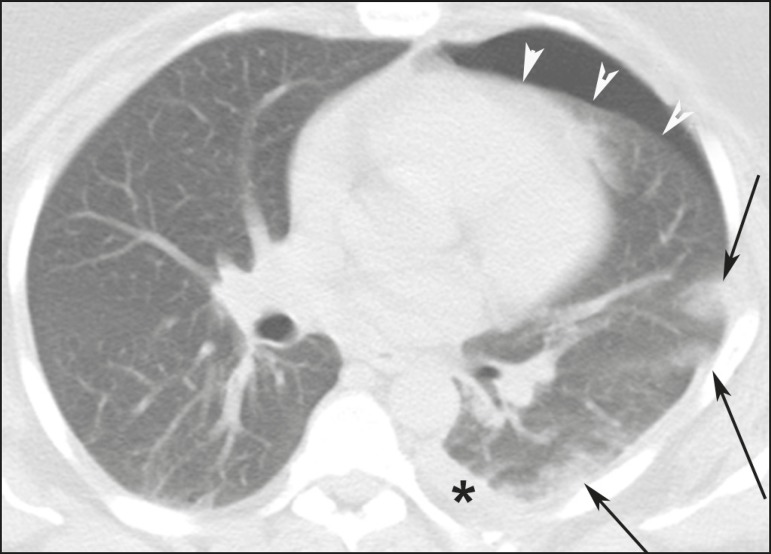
A 22-year-old male gunshot victim. Axial CT scan of the chest showing
